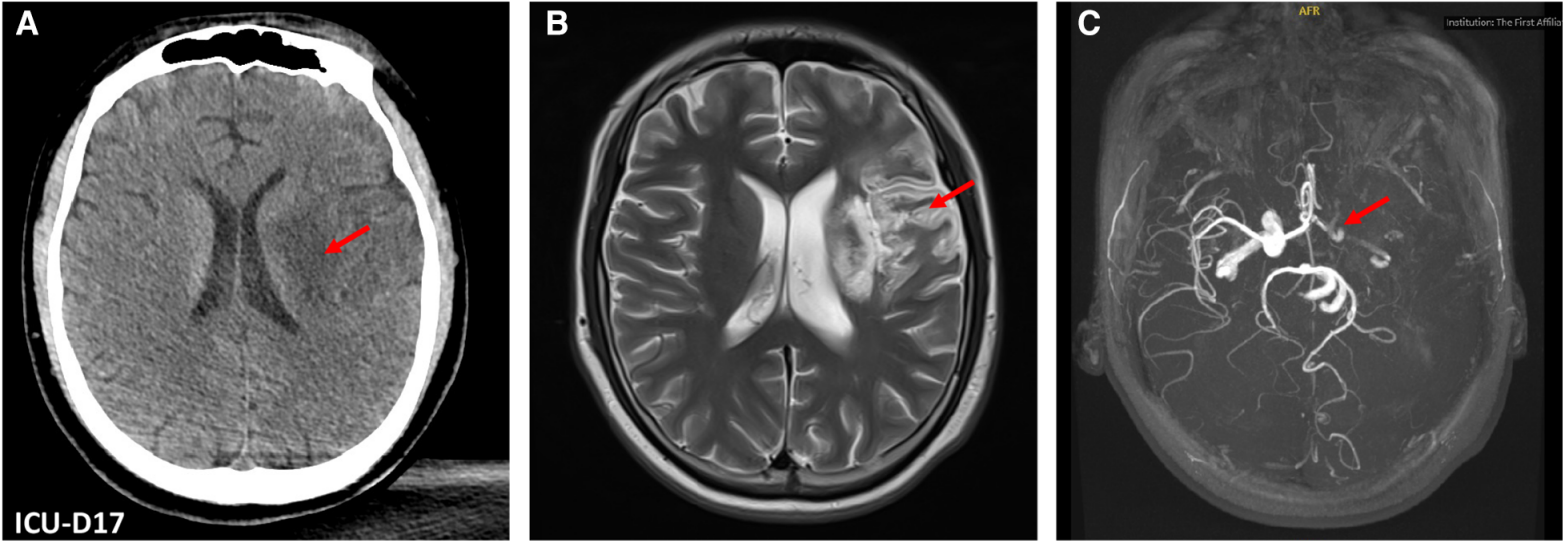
Brain computed tomography (CT) and magnetic resonance (MR) images. (A) Brain CT on ICU Day 17 revealed an acute infarction of a large area of left middle cerebral artery territory (red arrow). (B) The post-ICU discharge brain MRI showed hyperintense regions (red arrow) in the left frontotemporal and basal ganglia areas. (C) Brain MR angiography revealed diminished perfusion in the intracranial segment of the left cervical internal carotid artery left, along with occlusion of the left middle cerebral artery (red arrow).
